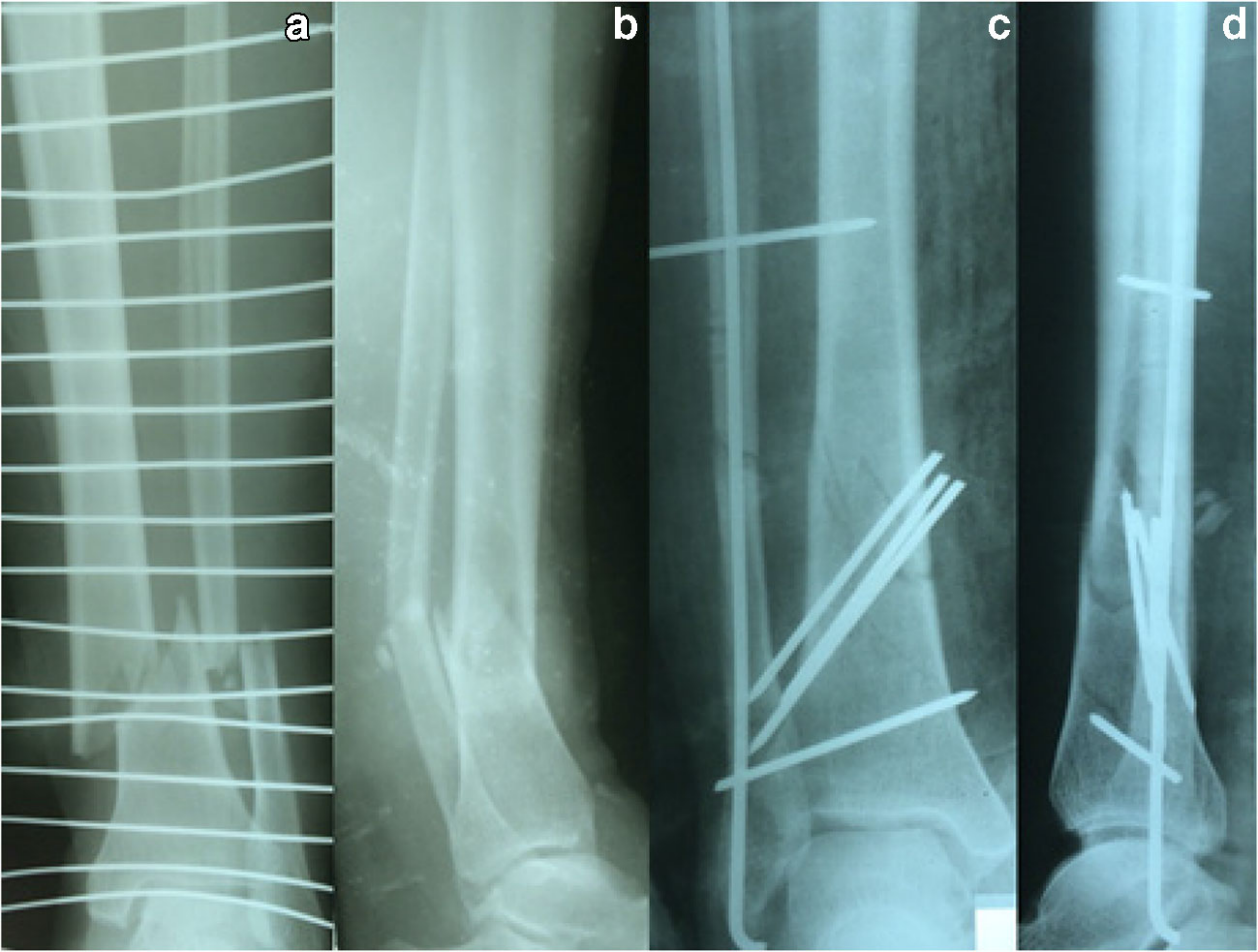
Implant not ready. A 34-year-old female patient sustained a III°B open tibial fracture AO 42 A2.3 by a road traffic accident. Anteroposterior and lateral X-ray of the fractured lower leg, III°B open (a and b). The patient was planned for external fixation as an emergency procedure. At the time of operation, no external fixator was ready in the OT. After debridement, we decided to fix the fracture with K-wires additionally to a cast. The K-wires should provide an anatomic reduction that the cast alone could not. The three intrafocal K-wires should hold the reduction of the tibia, the intramedullary K-wire align the fibula, and the two fibulotibial K-wires act as a frame fixation (c and d). As the K-wires would become loose and migrate, their intention is to hold the anatomic reduction as long as possible
